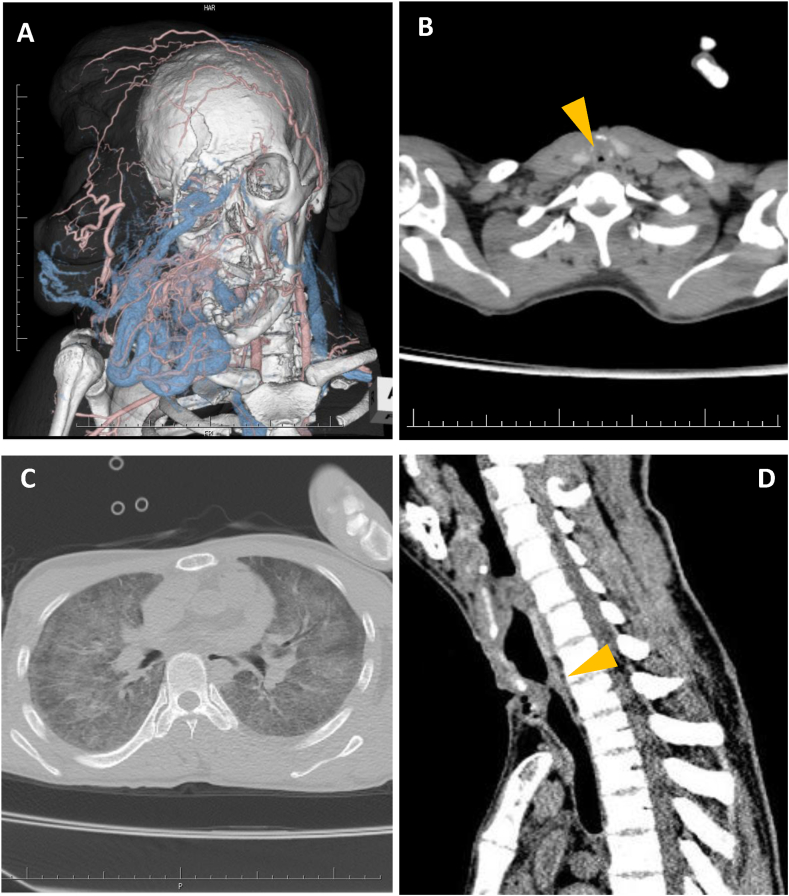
A. 3D CT image showed hypervascular mass that extended to the upper airway, causing severe deformation of the oral cavity and jaw, whereas the lower airway was intact. B. Postmortem CT revealed pinhole tracheal stenosis (arrowhead). C. Postmortem CT showed negative pressure pulmonary edema, suggesting asphyxia as the cause of death. D. Sagittal section of CT showed obstruction locating just beneath of tracheostomy site.
